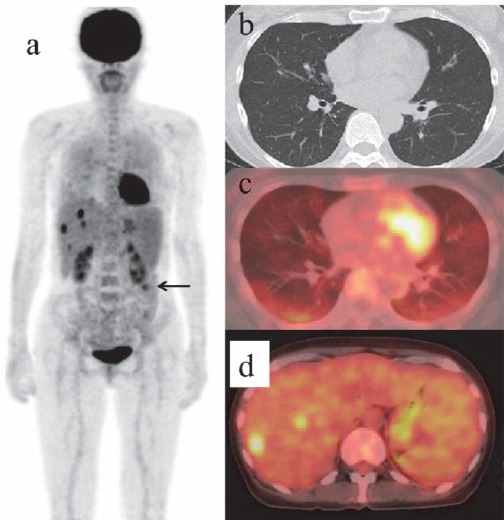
FDG-PET/CT (a, c, d) showed diffuse FDG uptake in the lung fields and in spleen and multiple hot spots in the liver. No abnormalities were detected in chest CT (b). The focal uptake inferior to the left kidney is physiological activity in small intestine (black arrow)
